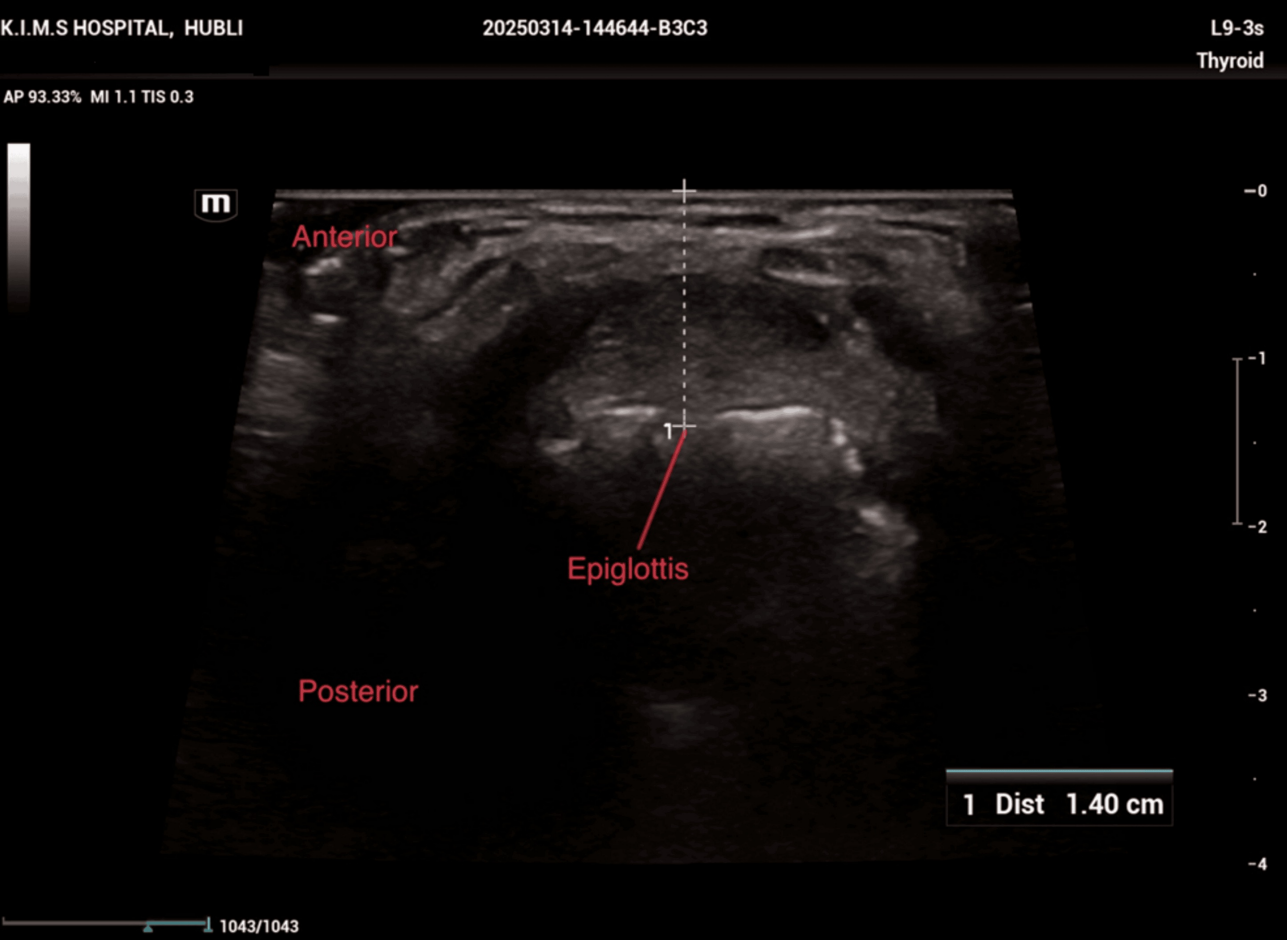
Ultrasonography measuring the skin-to-epiglottis distance
